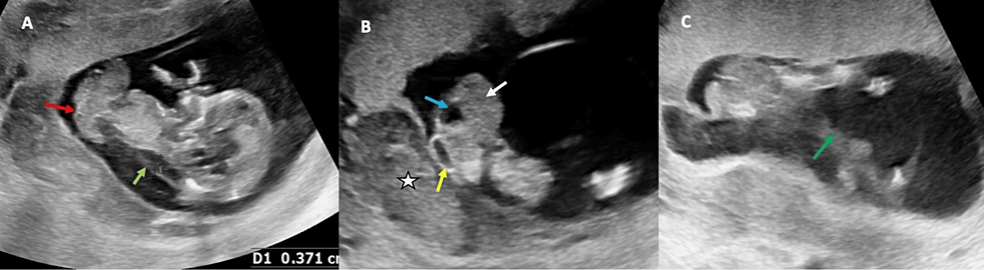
USG image showing abdominal wall defect with evisceration of abdominal organs (red arrow) and an increased nuchal translucency (light green arrow). A,B: the eviscerated organs included liver (white arrow), echogenic clumped bowel loops with stomach (yellow arrow), and urinary bladder (blue arrow); B: sub-chorionic hemorrhage (asterisk); C: hypoplastic right lower limb (green arrow). USG, ultrasound
